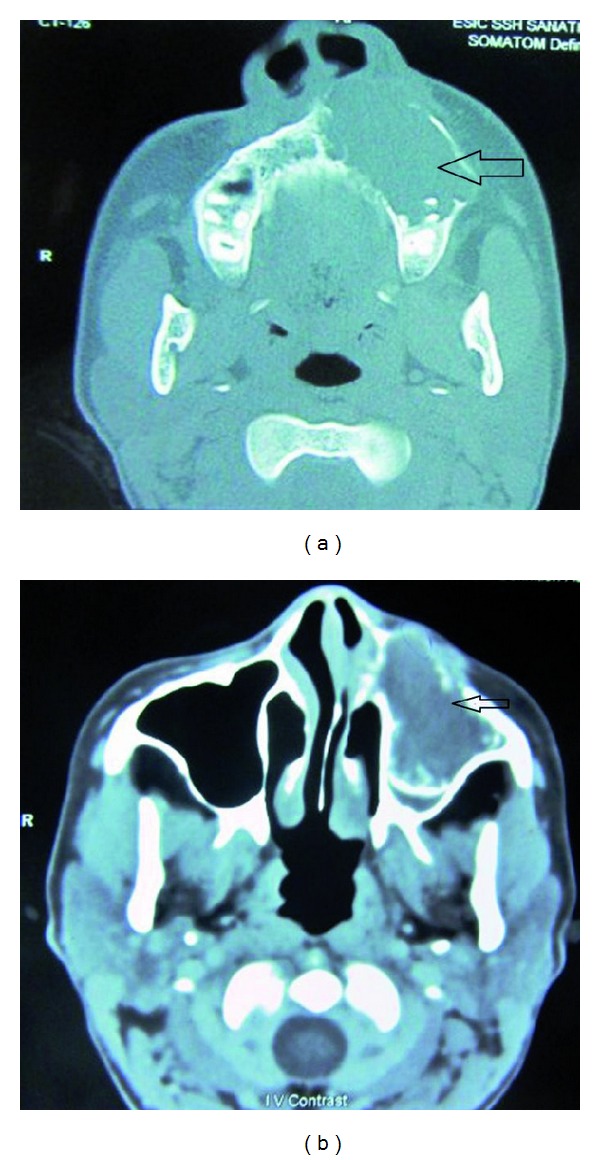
Axial CT Scan sections showing the lesion perforating the anterior wall of the antrum and its extension in to the nasal cavity.
